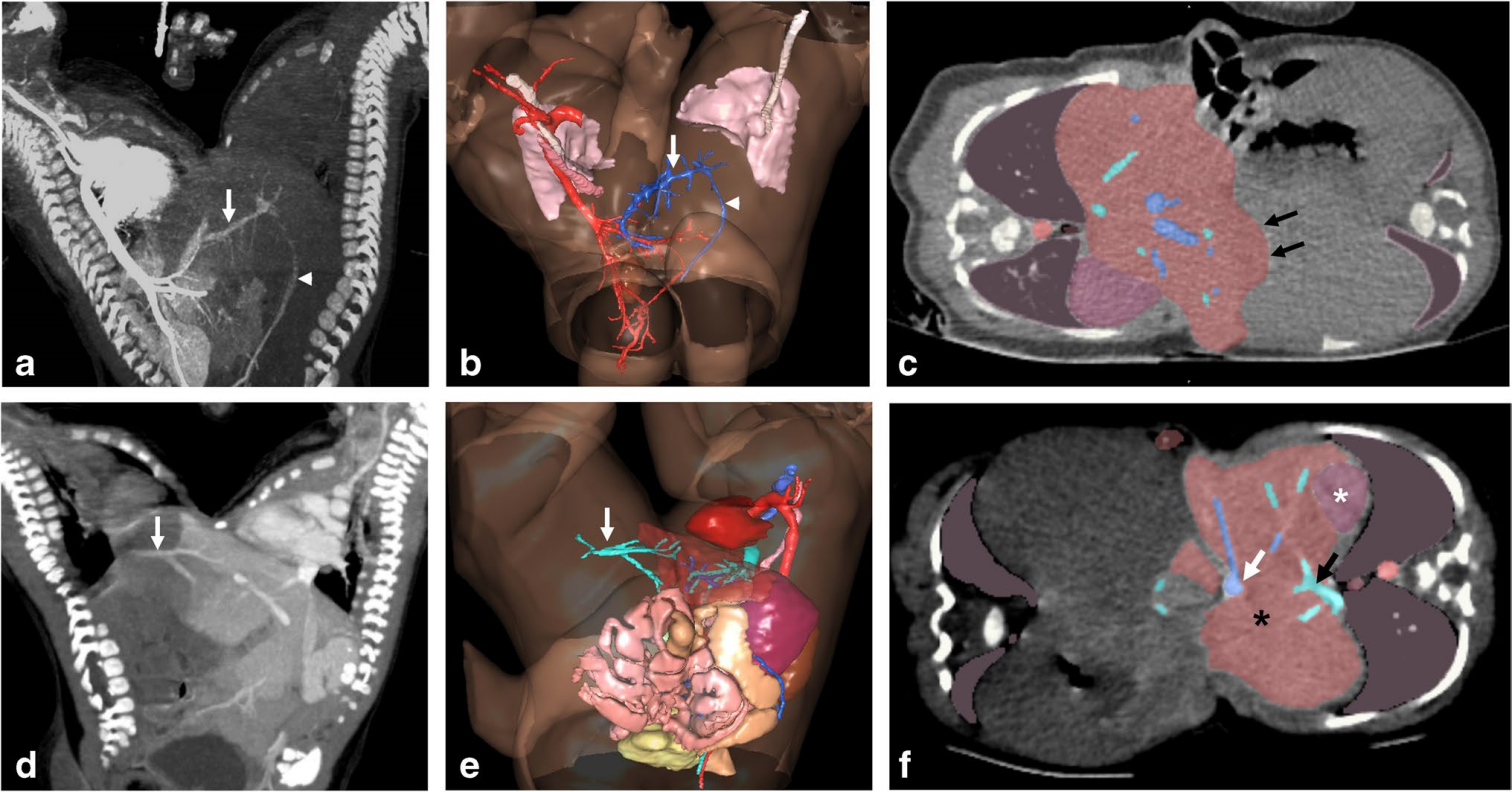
Computed tomography (CT) in 4-month-old ischiopagus conjoined twin boys following intravenous contrast injection of each twin on separate days. (a–c) are extracted following contrast injection of the right twin, while (d–f) are extracted following contrast injection of the left twin. a, b Maximum intensity projection (MIP) (a) and color-coded 3-dimensional (D) reconstruction) (b) show an abnormal venous channel (*arrow*) connecting the portal system of both twins with retrograde venous filling into the left twin *(arrowhead).*
